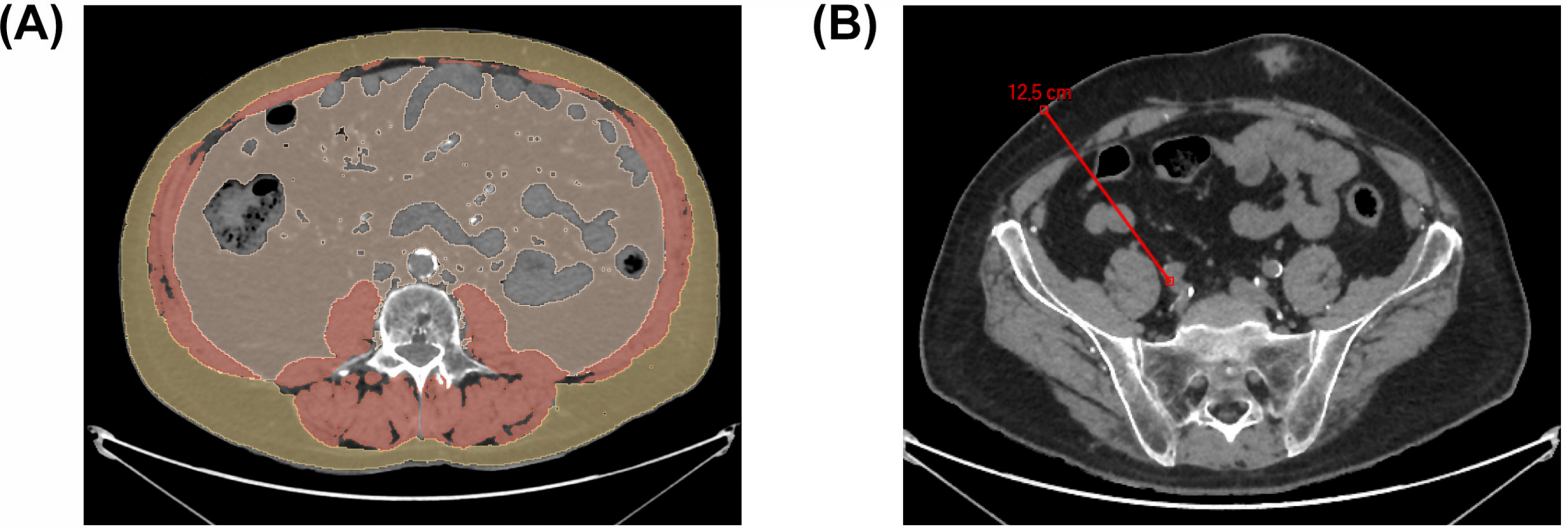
(A) Cross‐sectional computed tomography images in a kidney transplant recipient after muscle and fat segmentation at the level of the third lumbar vertebra. Skeletal muscle area (red) was identified by using computed tomography attenuation values of −29 to +150 Hounsfield units (HU). Subcutaneous fat area (yellow) was depicted by using attenuation values of −190 to −30 HU. For visceral fat area (orange), −150 to −50 HU attenuation values were used. (B) Vessel‐to‐skin (VSK) distance measurement on abdominal CT scan. VSK was defined by the distance between the anterior edge of the iliac vein and the skin, passing through the semilunar line.
